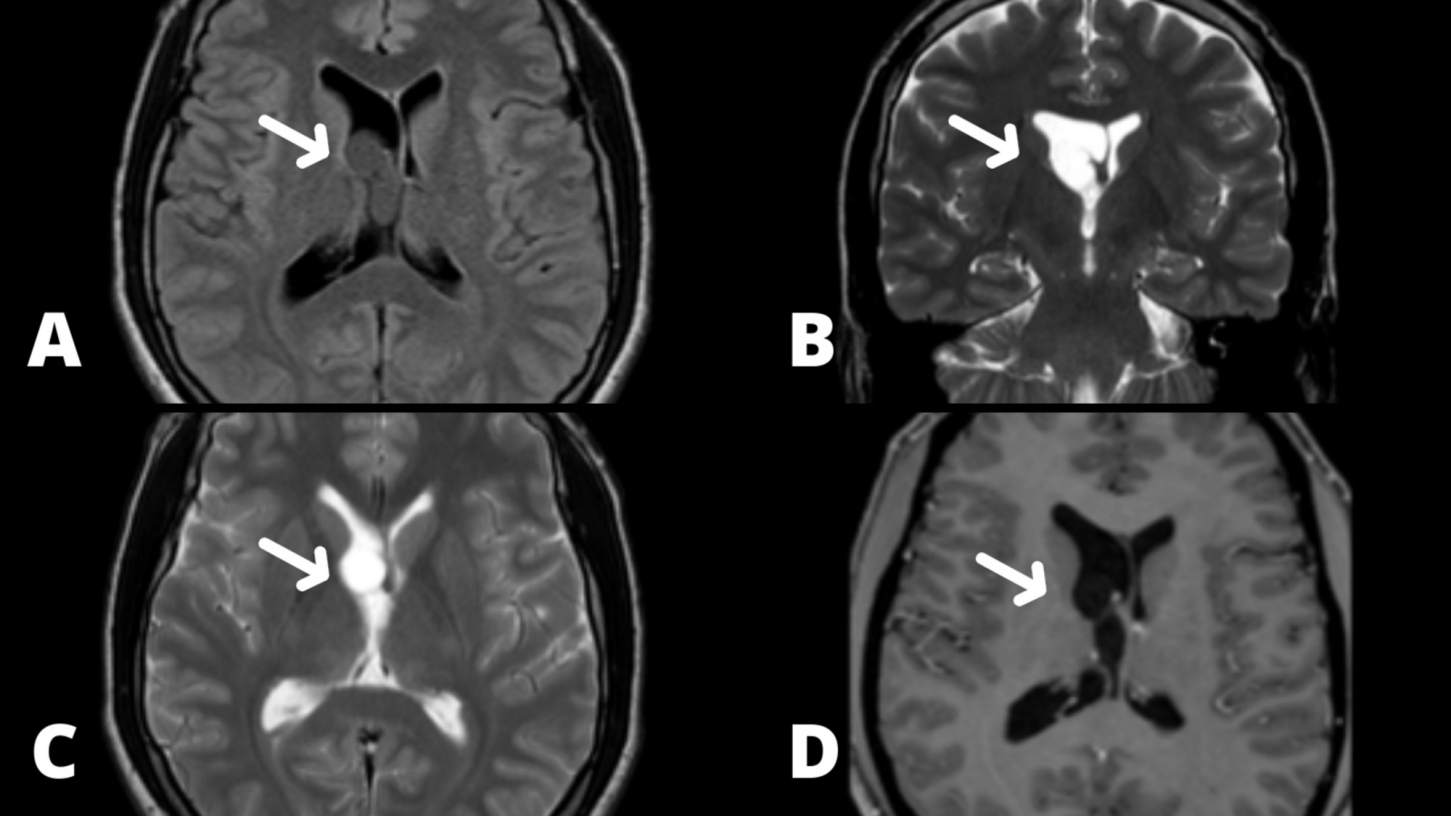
Preoperative axial MRI scan. The images A-D show an enlargment of the right lateral ventricle due to a cyst blocking the right foramen of Monro.
